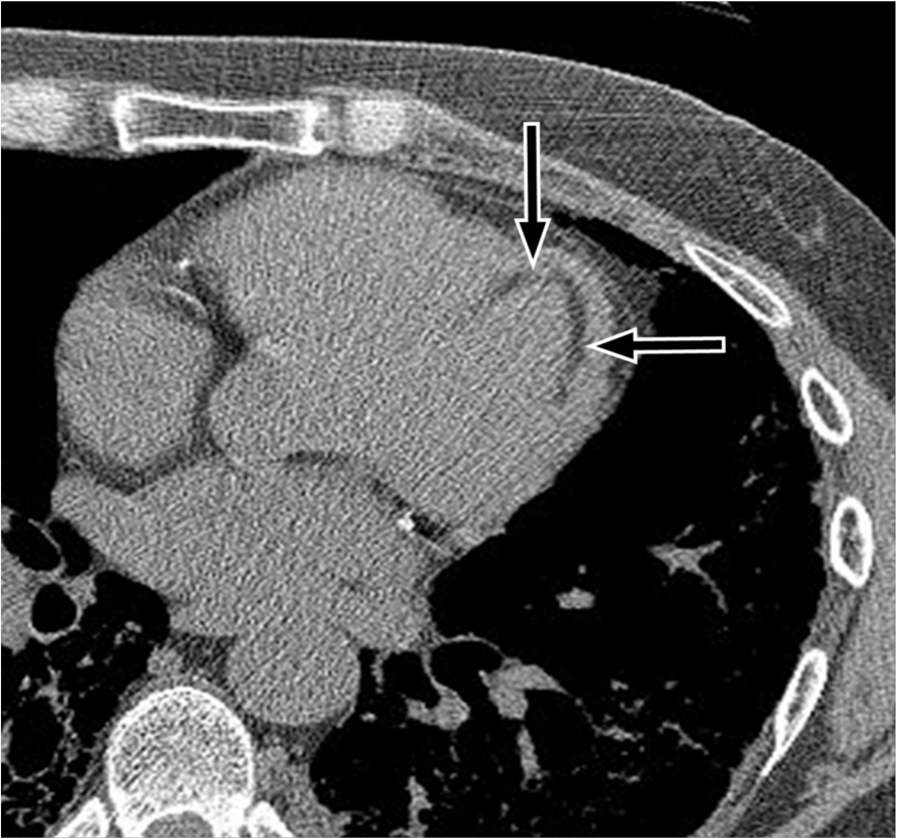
Axial image demonstrates fat replacement in an apical left ventricular infarct on a patient being evaluated for chronic dyspnea by high resolution computed tomography (HRCT)
